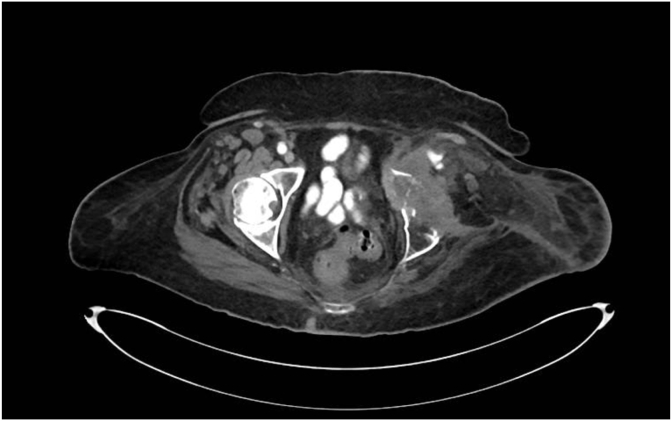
Axial CT pelvis demonstrating enlarging left acetabular mass encasing the left external iliac artery and involving the left sciatic nerve.
